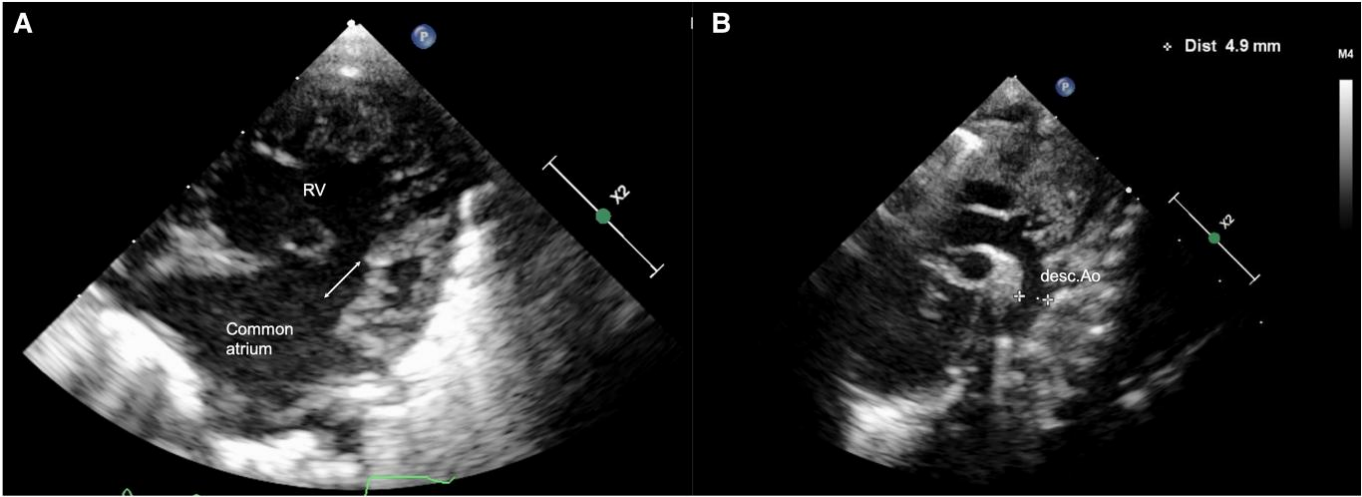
Echocardiogram findings on admission showing four-chamber view (*A*), aortic arch (*B*). White arrow indicates suspected ventricular septal defect. RV, right ventricle; desc.Ao, descending aorta.
